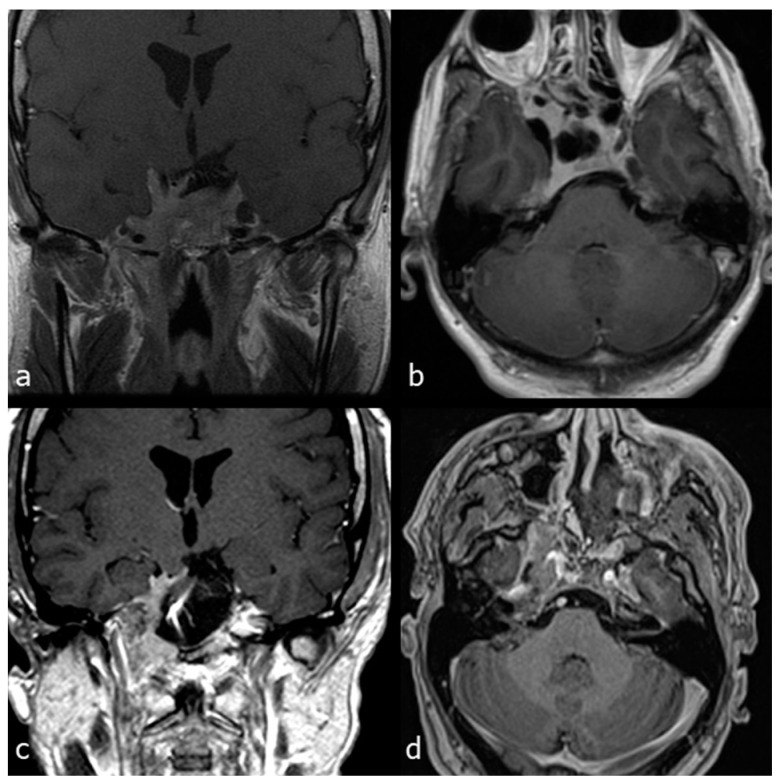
Case 3: preoperative and postoperative MRI scans of a recurrent giant non-secreting pituitary adenoma, previously operated through transcranial and then operated on by a combined EETC and CTM approach. (a) preoperative MRI scan coronal view. (b) preoperative MRI scan, sagittal view. (c) postoperative MRI scan, coronal view. (d) postoperative MRI scan, sagittal view.
